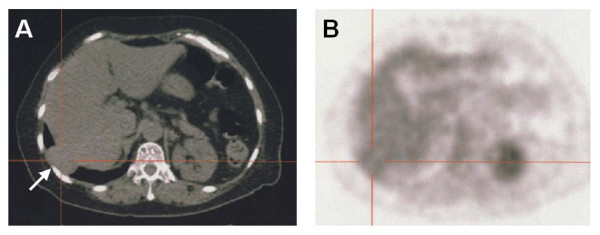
CT and corresponding PET findings of the tumor. A CT before the operation for liver herniation showed a 3.7 × 2.7 cm solid tumor (arrow). B The corresponding PET scan revealed the FDG uptake (SUV max was 3.1), which was equal to the level in liver parenchyma, and contiguous with the liver.
